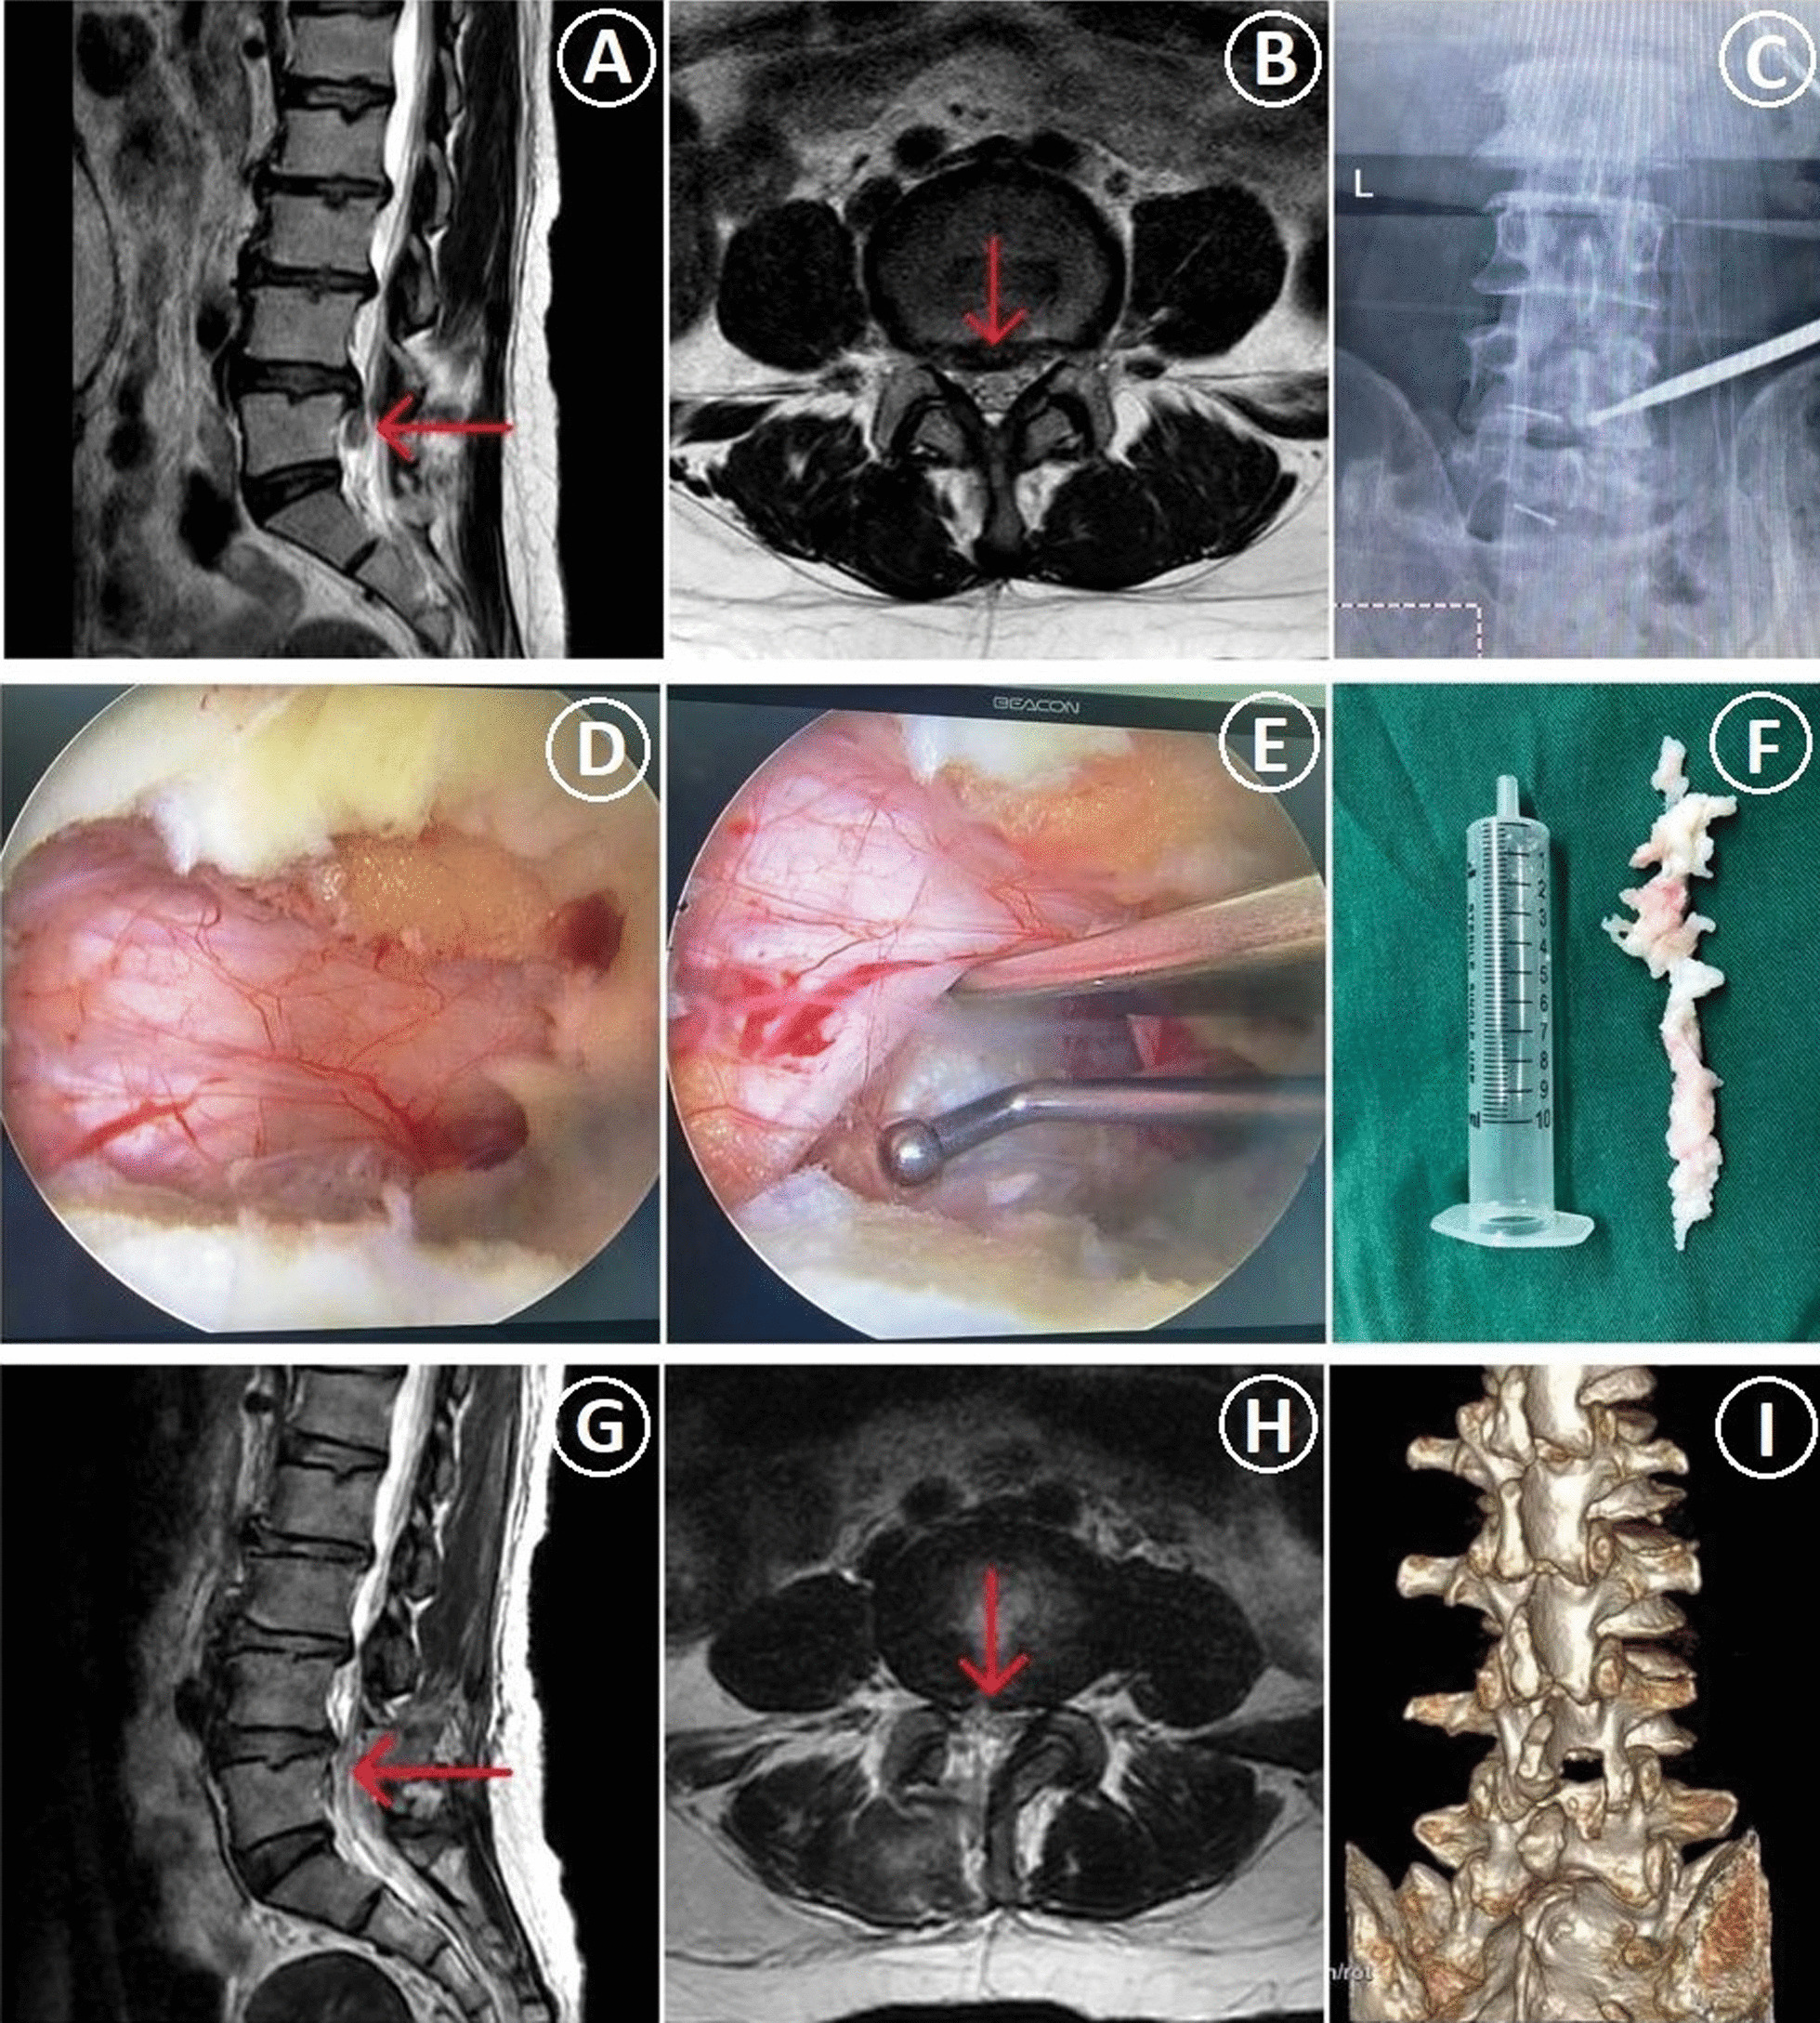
A 48-year-old female was diagnosed preoperatively with high-grade migrated LDH at the L4–5 level and underwent discectomy by OSE. A, B The preoperative T2-weighted MR images showed that the migrated intervertebral disc was located in zone 4. C Intraoperative anteroposterior fluoroscopy was used to confirm the responsible segment. D, E Images under the endoscope. F The nucleus pulposus tissue. G, H The postoperative T2-weighted MR images showed that the migrated intervertebral disc was completely removed. I The 3D CT showed that partial bone defected
